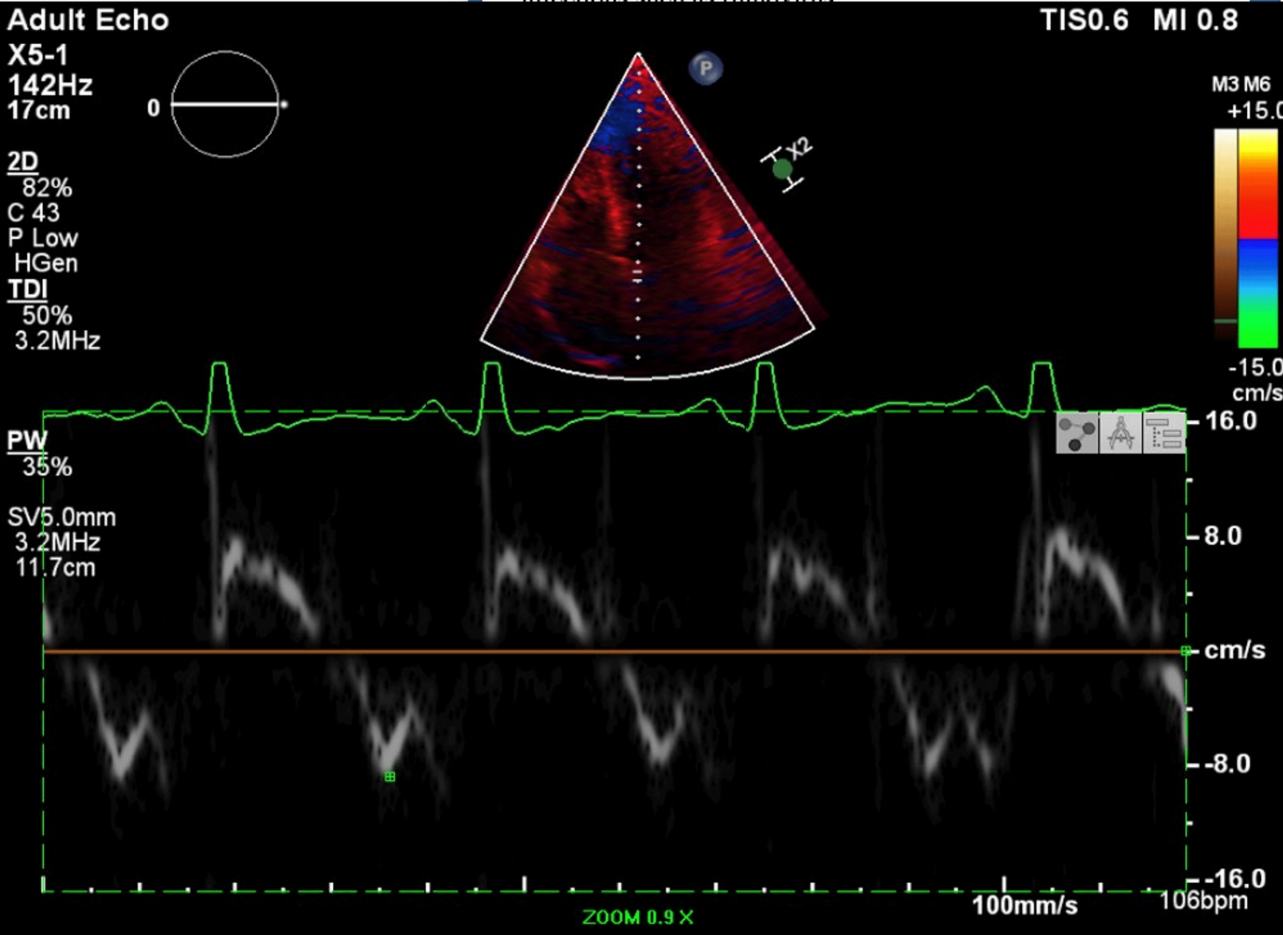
Tissue Doppler imaging from the apical four-chamber view at the septal mitral annulus The early diastolic velocity (e') measures approximately 10 cm/s
